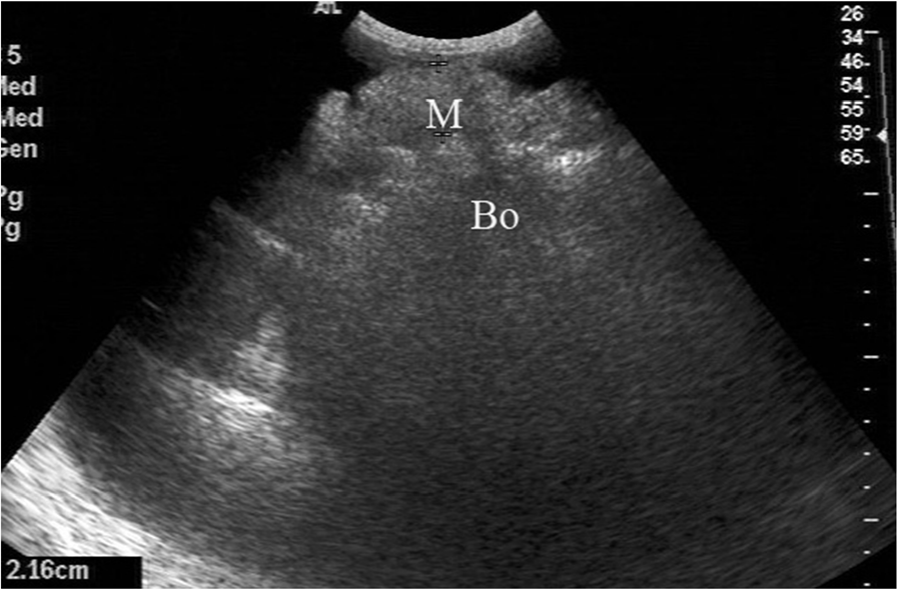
Data for a 46-year-old woman. Ultrasonography showed a thickening of the visceral peritoneum (M) with homogeneous hyperechoes; this thickening was pathologically diagnosed as malignant peritoneal mesothelioma.
